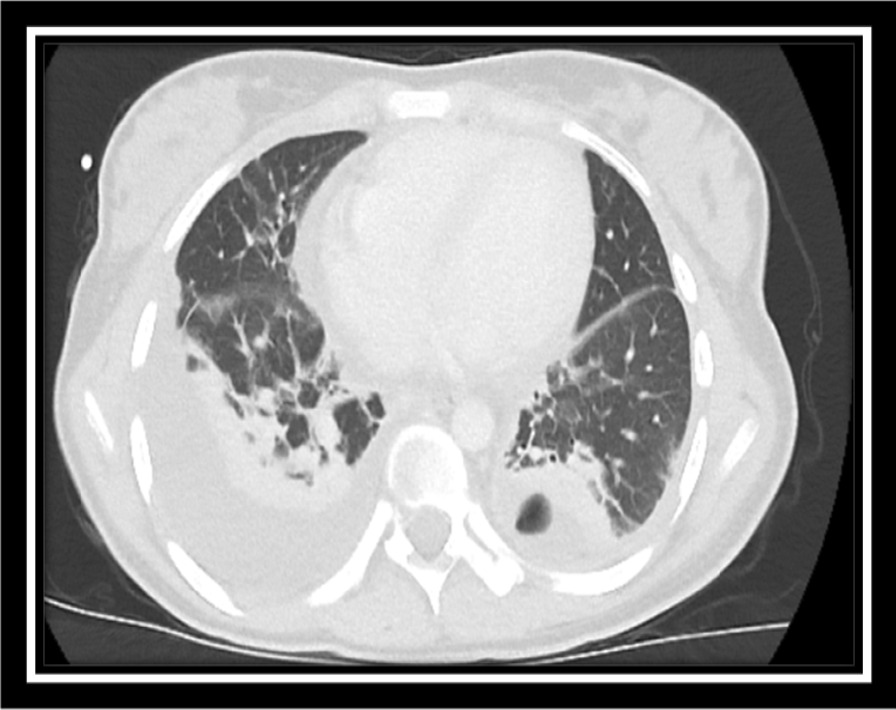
Thoracic computed tomography, lung window: pleural fluid with dense content
